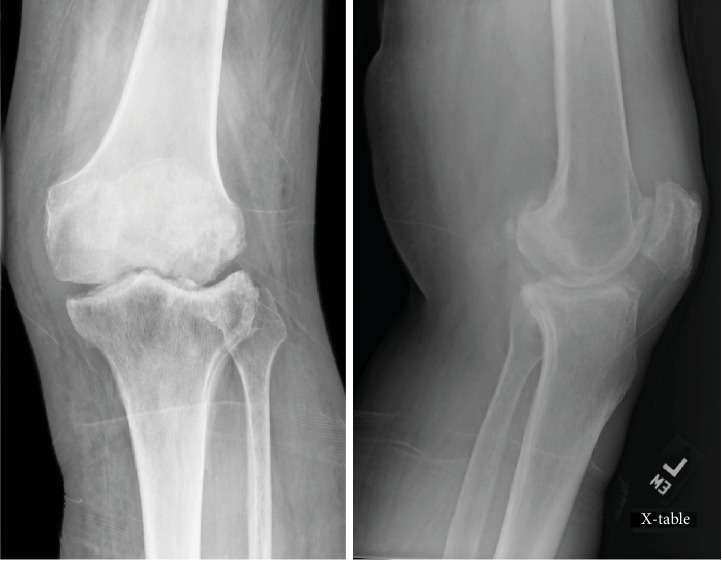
Left knee anteroposterior (AP) and lateral plain films status post open irrigation and debridement.
